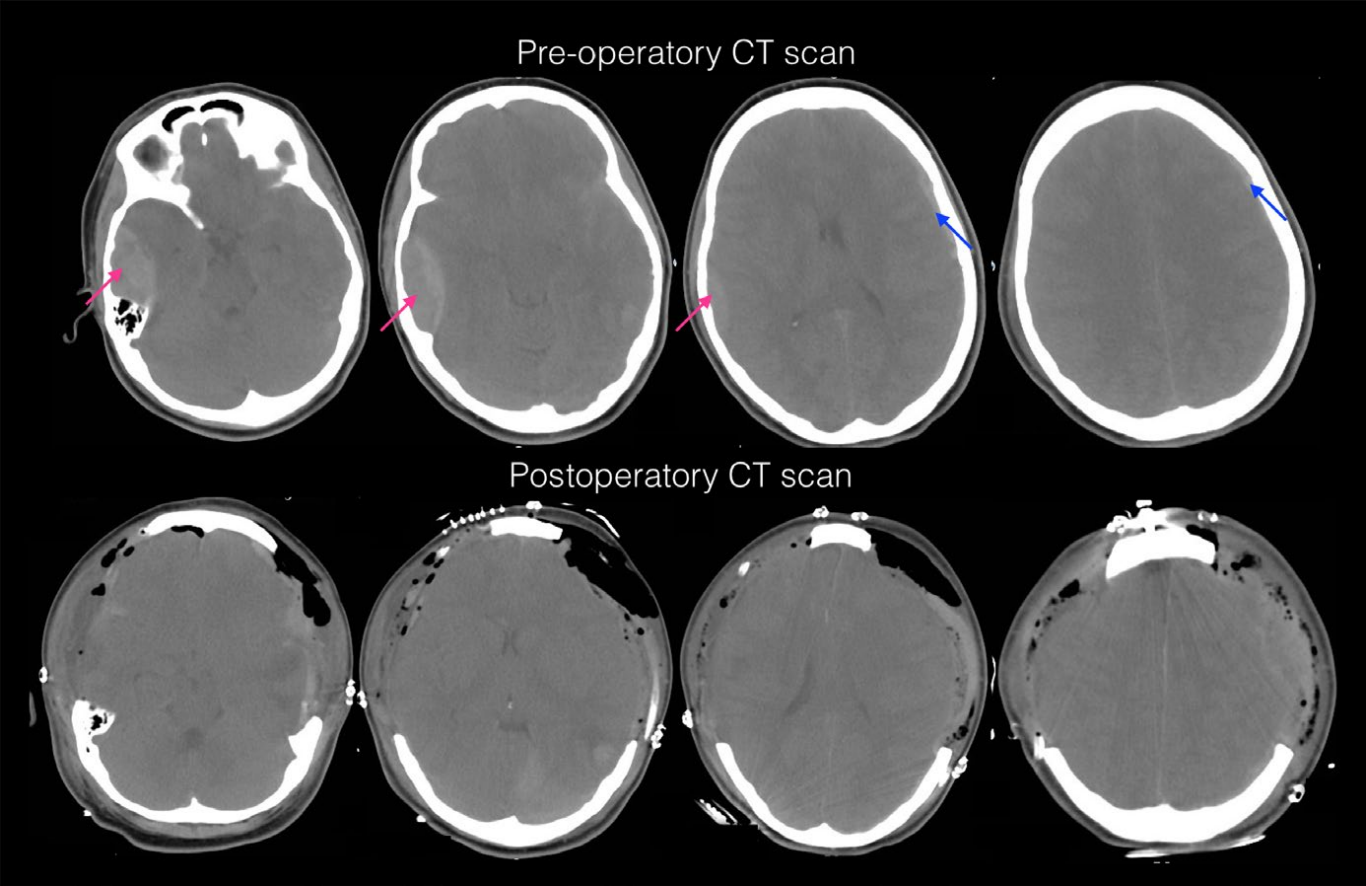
Case 1: Admission CT scan shows a right frontotemporal epidural hematoma that is 2.5 cm in diameter (red arrow), a small left fronto‐parietotemporal subdural hematoma (blue arrow), a left traumatic subarachnoid hemorrhage, and small left brain contusions. Additionally, compressed cisterns can be observed. The CT scan 24 h after surgery. BFTP‐DC was performed. Pneumocephalus and herniation of the brain tissue through the craniectomies can be observed
